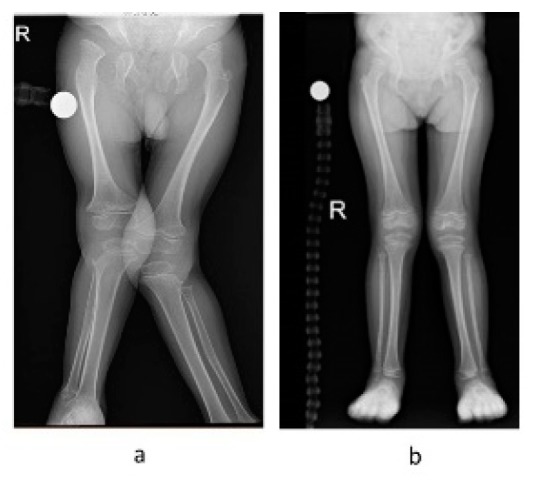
Radiographic pictures of the lower extremities of patients 1 and 2: (a) patient 1 (84 months); (b) patient 2 (48 months).
